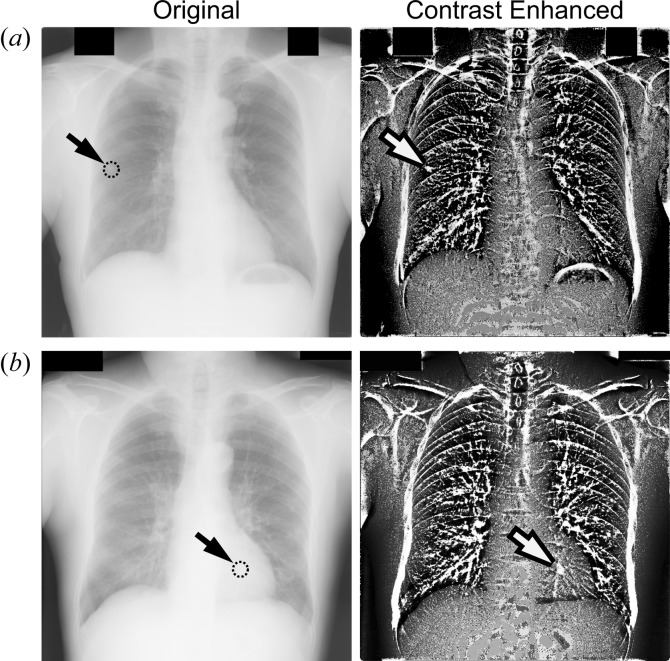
Enhancement of chest radiographic images by the proposed method. Left-hand panels: original chest radiographic images [(*a*) JPCLN80, (*b*) JPCLN152]. The arrow in each image indicates a nodule. Right-hand panels: contrast enhanced images obtained by the proposed method.
